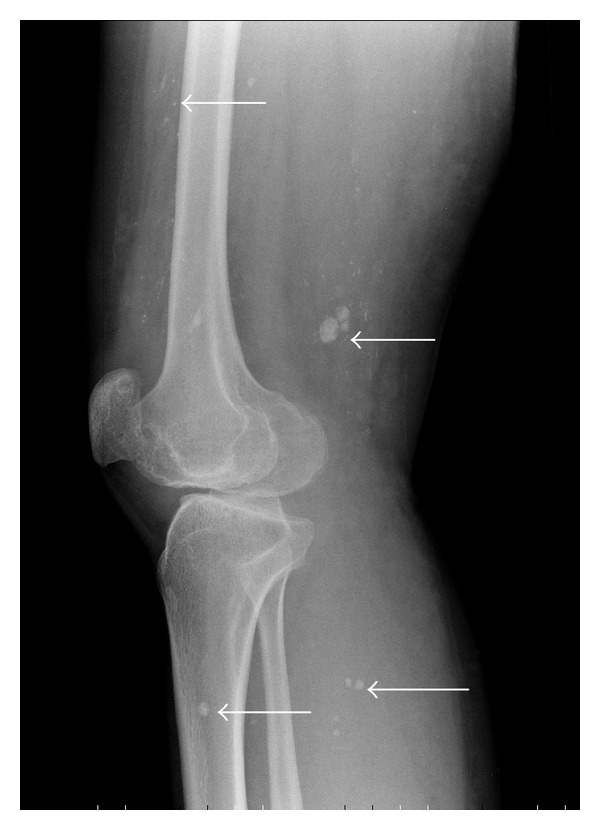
Lateral radiograph of the knee demonstrating multiple venous calcifications (phleboliths) (arrows).
